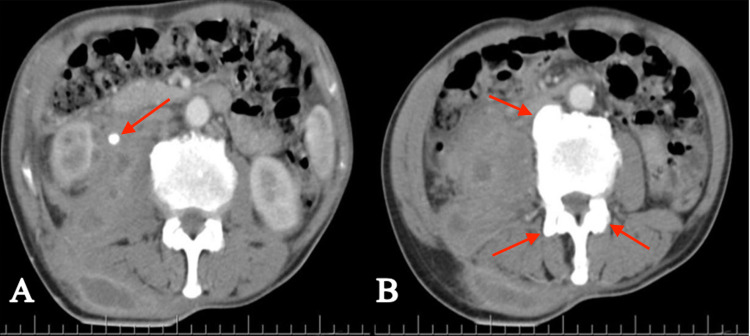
Abdominal CT scan with contrast A) Right kidney with markedly increased size; the presence of a 7.5-mm and 409-UH lith is apparent in the proximal third of the right ureter. Adjacent to it, an image compatible with a collection of heterogeneous aspects is evident, which compromises the right paracolic gutter and muscular planes (right oblique muscle, psoas muscle, and paravertebral muscles). The collection measures approximately 17.7x9.8x7.9 cm and has a volume of 712 cc. B) Degenerative changes at the level of the dorsolumbar area, indicated by the presence of osteophytes CT: computed tomography
